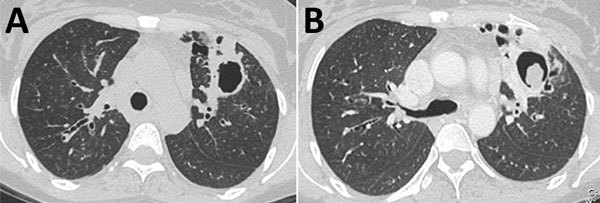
Computed tomography images of a patient with chronic pulmonary aspergillosis. A) Left upper lung lobe thick-walled cavity, showing associated pleural thickening. B) Same patient several months later, demonstrating progression of cavitation with increased pericavitary consolidation and formation of a fungal ball within the cavity. Aspergilloma formation is a late feature of chronic pulmonary aspergillosis.
